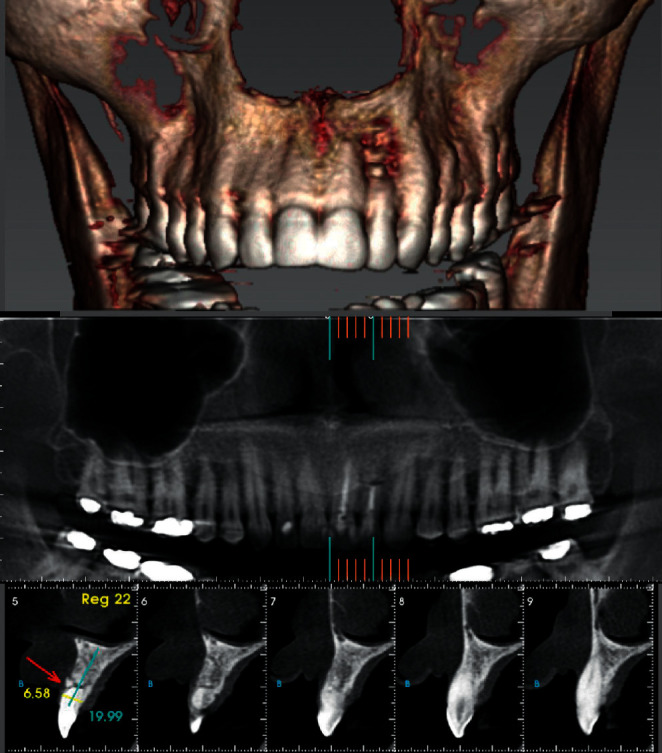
CBCT images of the periapical lesion of tooth #22 after 12 months.
